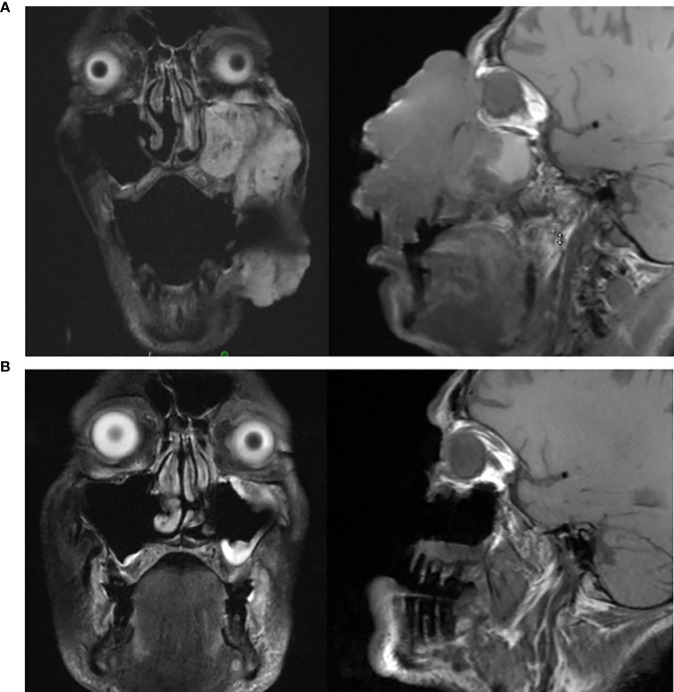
(A, B): (A) (top): MRI of head prior to initiating cemiplimab; (B) (bottom): MRI of head following completion of cemiplimab.
